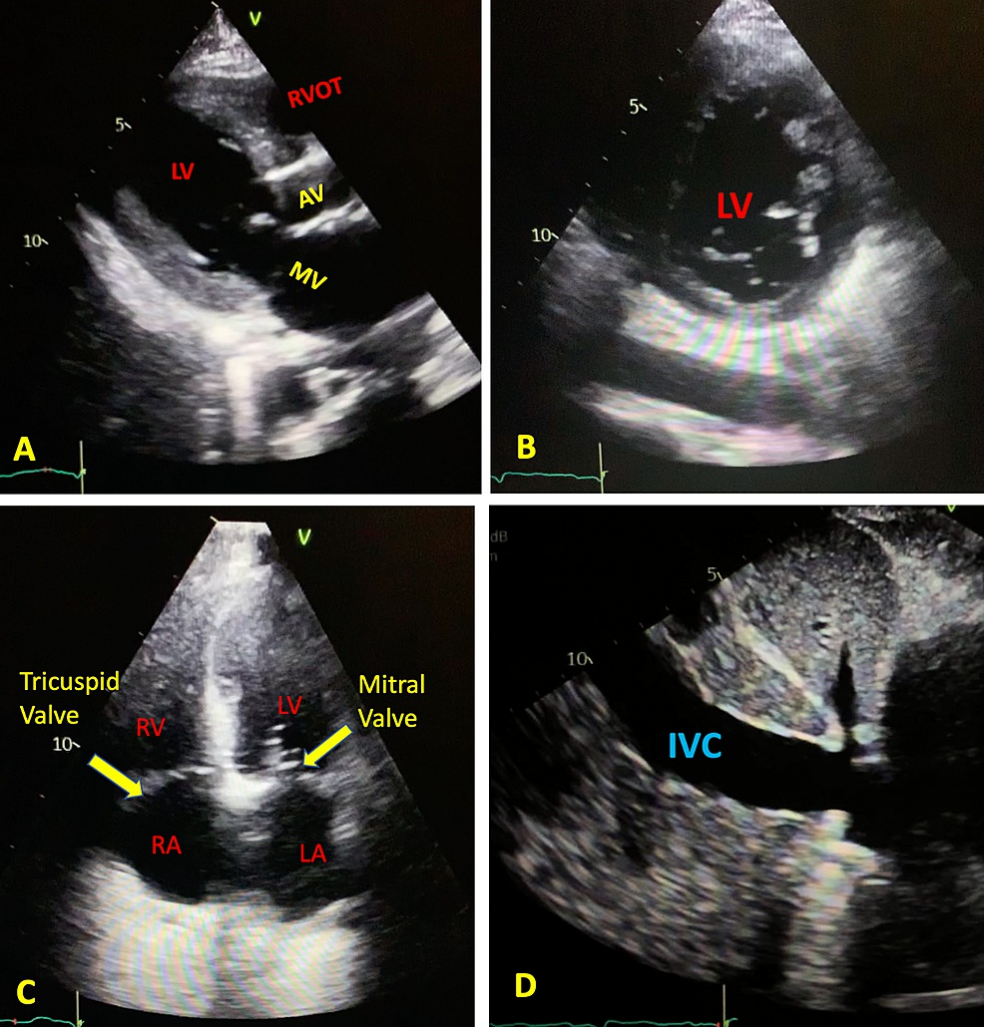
Cardiac Echo Cardiogram 2D - [A] Parasternal long axis view - LV [B] Parasternal short axis view [C] Four Chamber View [D] Subcostal view LA - Left Atrium, LV - Left Ventricle,  RA - Right Atrium, RV - Right Ventricle, AV - Aortic Valve, MV - Mitral Valve, RVOT - Right Ventricular Outflow Tract, IVC - Inferior Vena Cava
